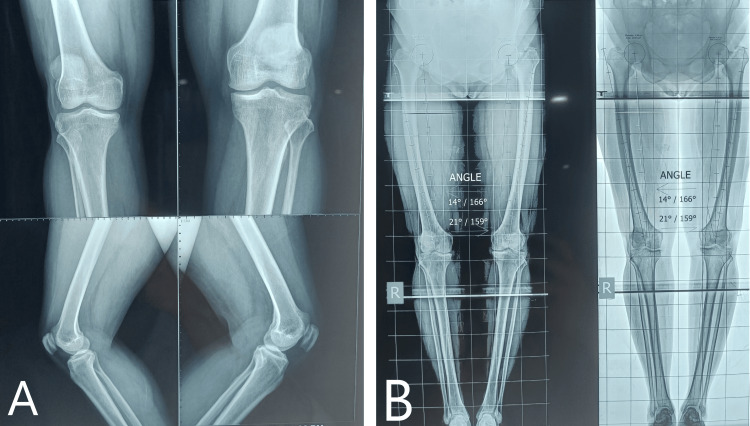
A) Pre-operative X-ray in weight bearing anteroposterior and lateral views. B) Orthoscannogram of both lower limbs in weight bearing standing position showing varus angulation.
